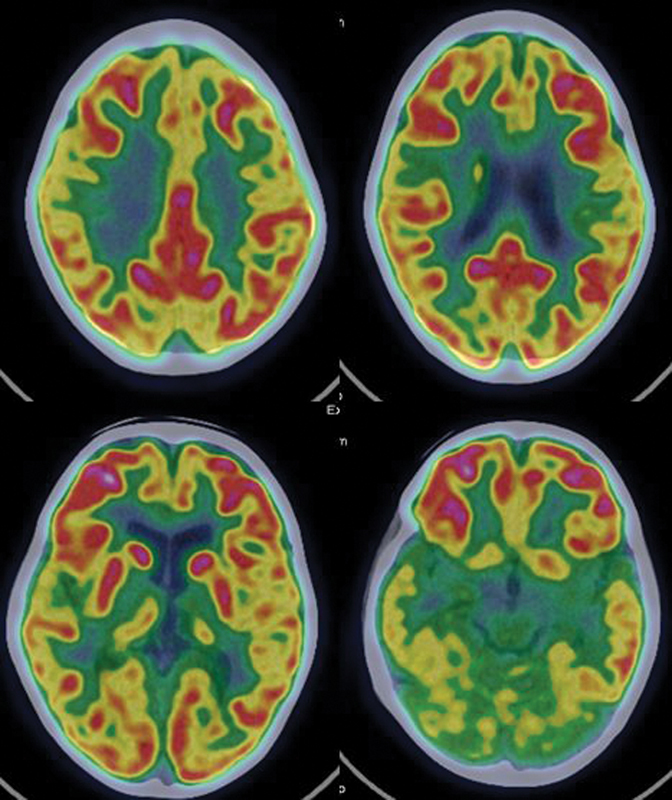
A 10-year-old right-handed boy, with normal milestones presented with focal seizures in sleep since 6 years of age associated with behavioral disturbances and poor scholastic performance. His IQ was 62 and CBCL score was 16. There were no focal neurological deficits. EEG showed left centroparietal predominance during the ESES. PET-CT showed hypometabolism in both thalami (left > right) with relatively normal metabolism in rest of the brain parenchyma. CBCL, child behavior checklist; CT, computed tomography; EEG, electroencephalography; ESES, electrical status epilepticus in sleep; IQ, intelligence quotient; PET, positron emission tomography.
